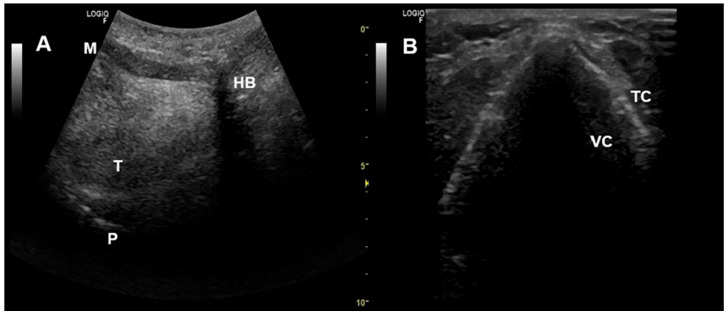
Upper airway ultrasound. (A) Oropharyngeal view. (B) Glottic view. HB—Hyoid bone, M—Mentum, P—Palate, T—Tongue, TC—Thyroid cartilage, VC—Vocal cords.
